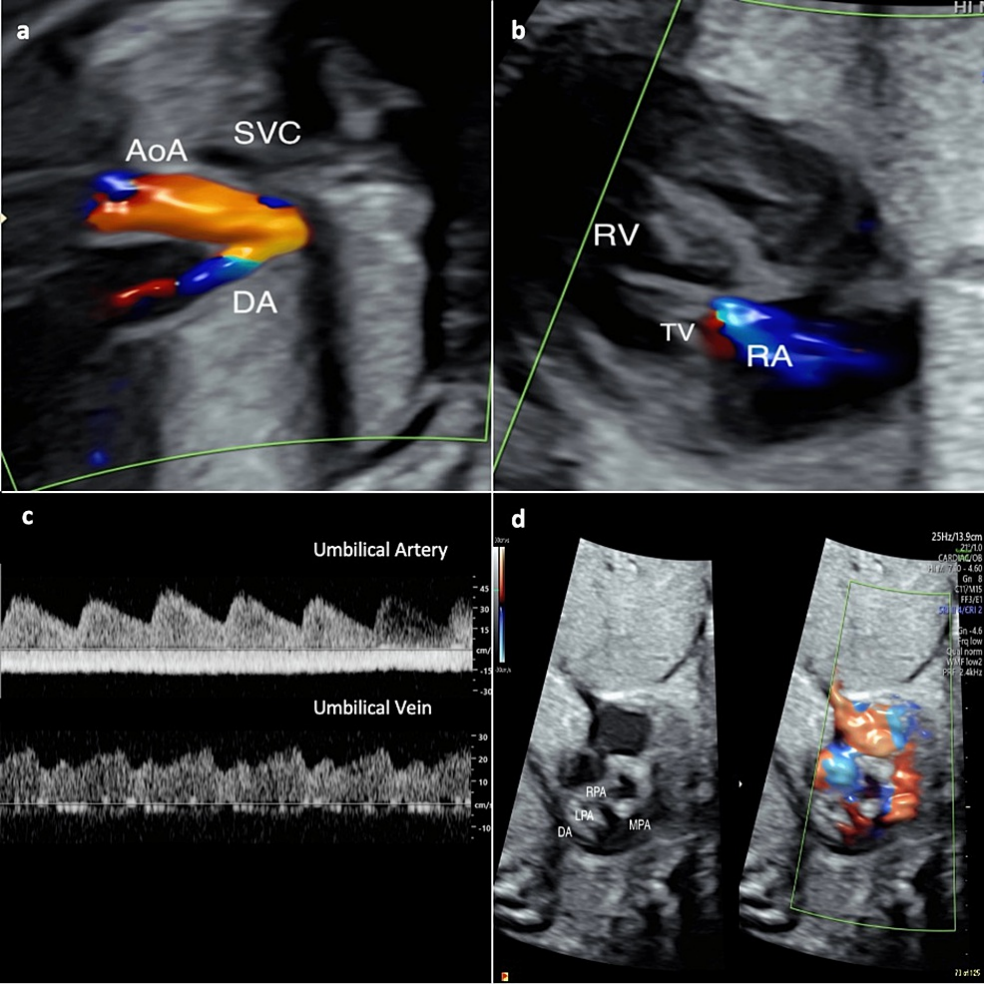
Fetal echocardiogram showing severe ductal arch constriction (a), moderate tricuspid regurgitation (b), normal umbilical vein and ductus venosus Dopplers (c), and follow-up examination showing widely patent ductus arteriosus (d).
